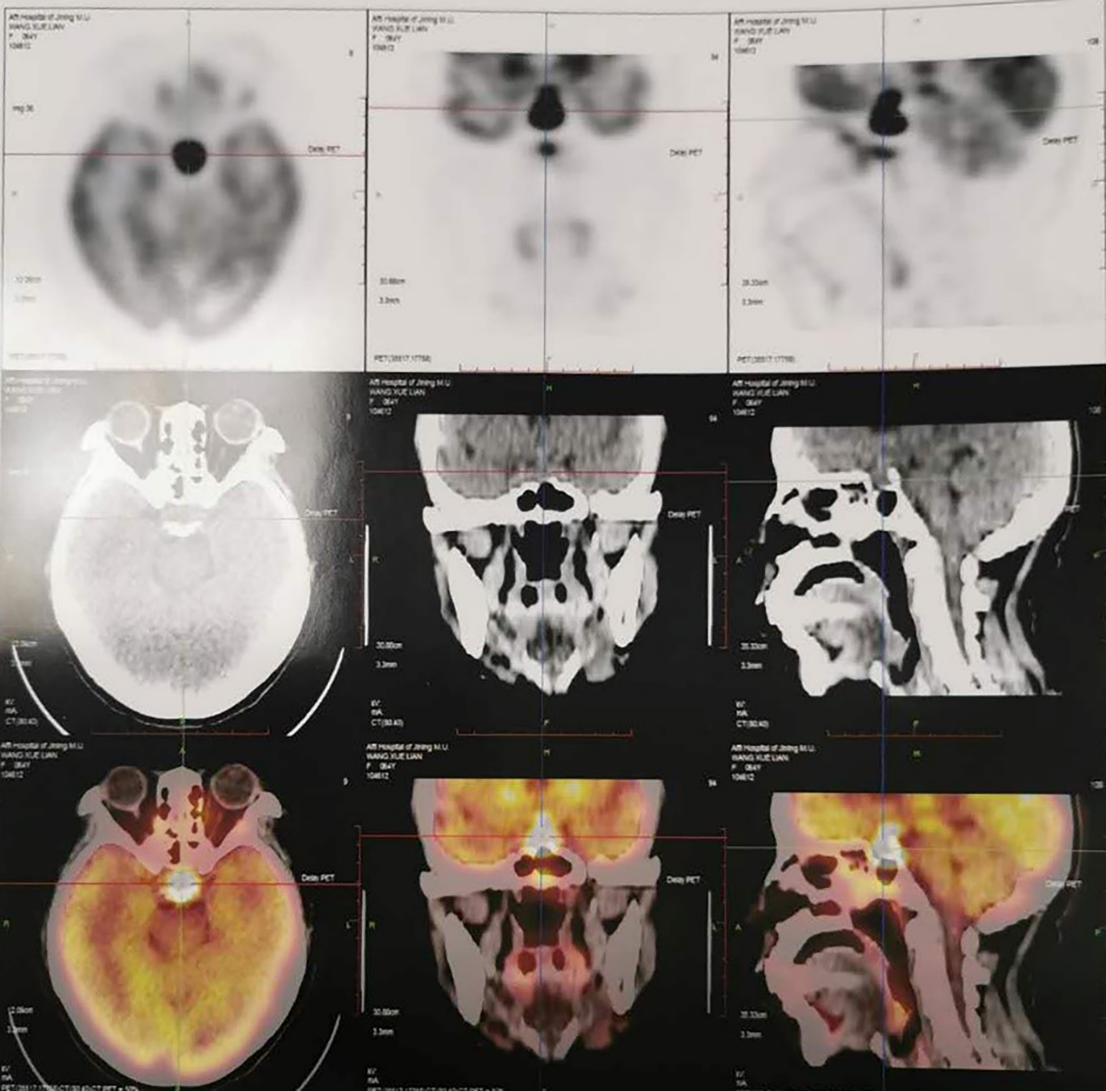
PET-CT one month ago showed the mass (2.3 × 2.0 cm) in pituitary gland with increased FDG uptake and SUVmax of 75.7 in Case 2.
